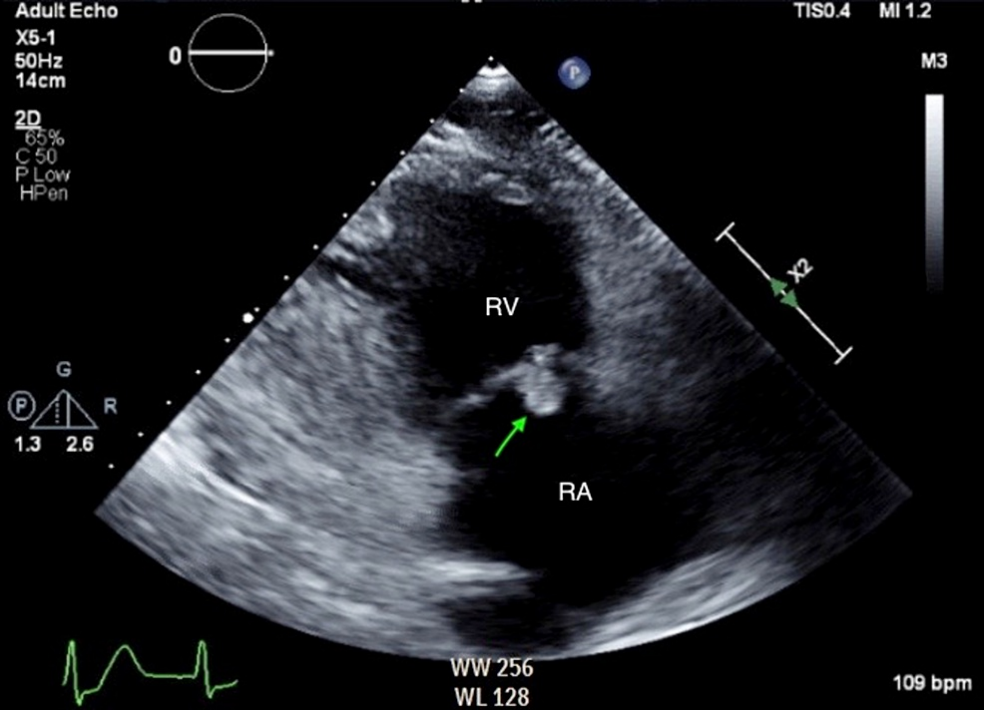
Transthoracic echocardiogram, right ventricle inflow view Shows a right atrial vegetation or mass (arrow) that looks like tricuspid valve vegetation. RA: right atrium, RV: right ventricle.
